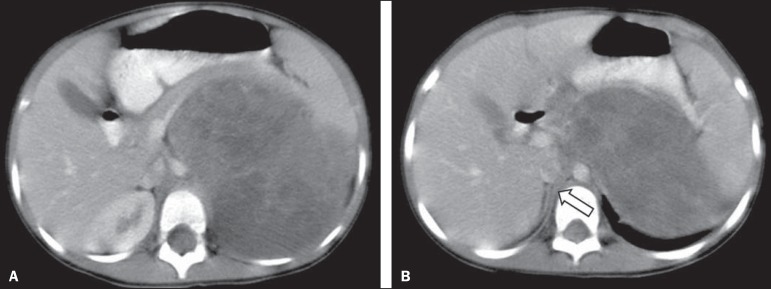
CT scans with intravenous and oral contrast of patients with masses that
